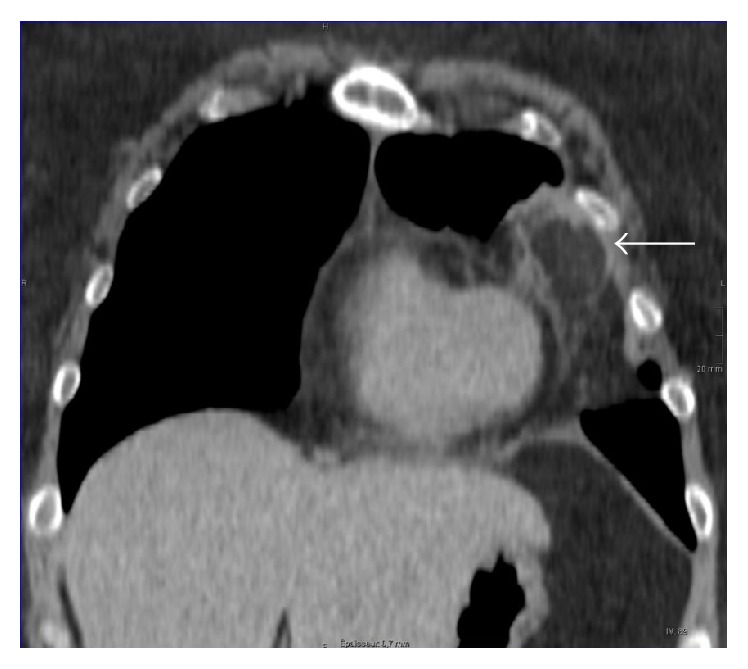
Coronal reformatted CT showing no connection between the pericardial mass and infradiaphragmatic fatty tissues.
